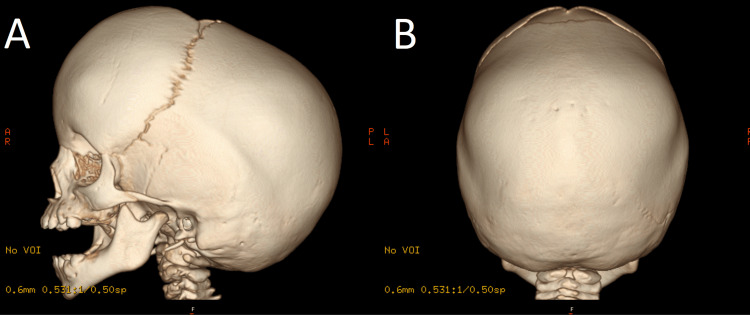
Cranial computed tomography performed at 18 months. (A) Increased anteriorposterior diameter (dolichocephaly). Also, left temporoparietal suture has decreased patency. (B) Sagittal and lambdoid sutures are closed bilaterally.
